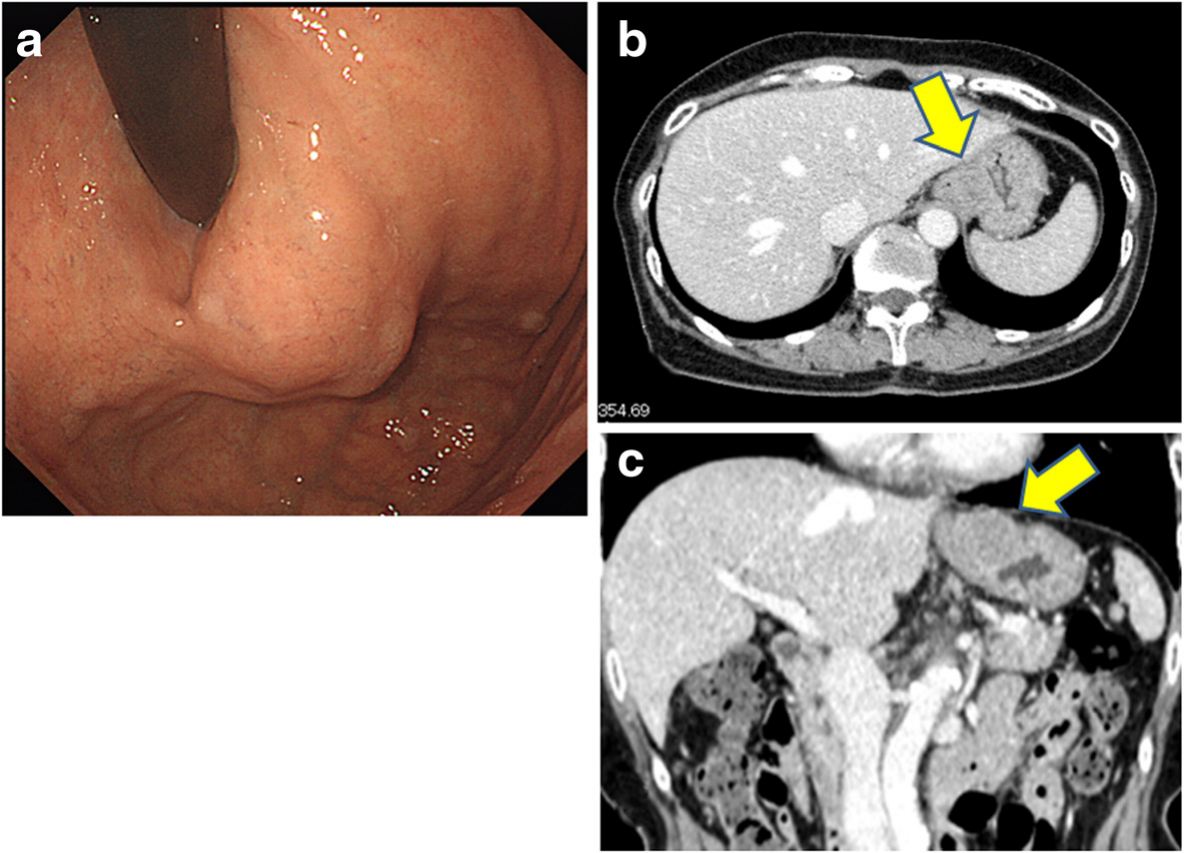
a Gastroduodenal endoscopy revealing the presence of a submucosal tumor at the greater curvature of the esophagogastric junction. b, c The enhanced computed tomography (CT) scan shows a smooth-outlined hypervascular solid mass (23 mm × 20 mm) in the gastric wall at the esophagogastric junction (*arrow*)
